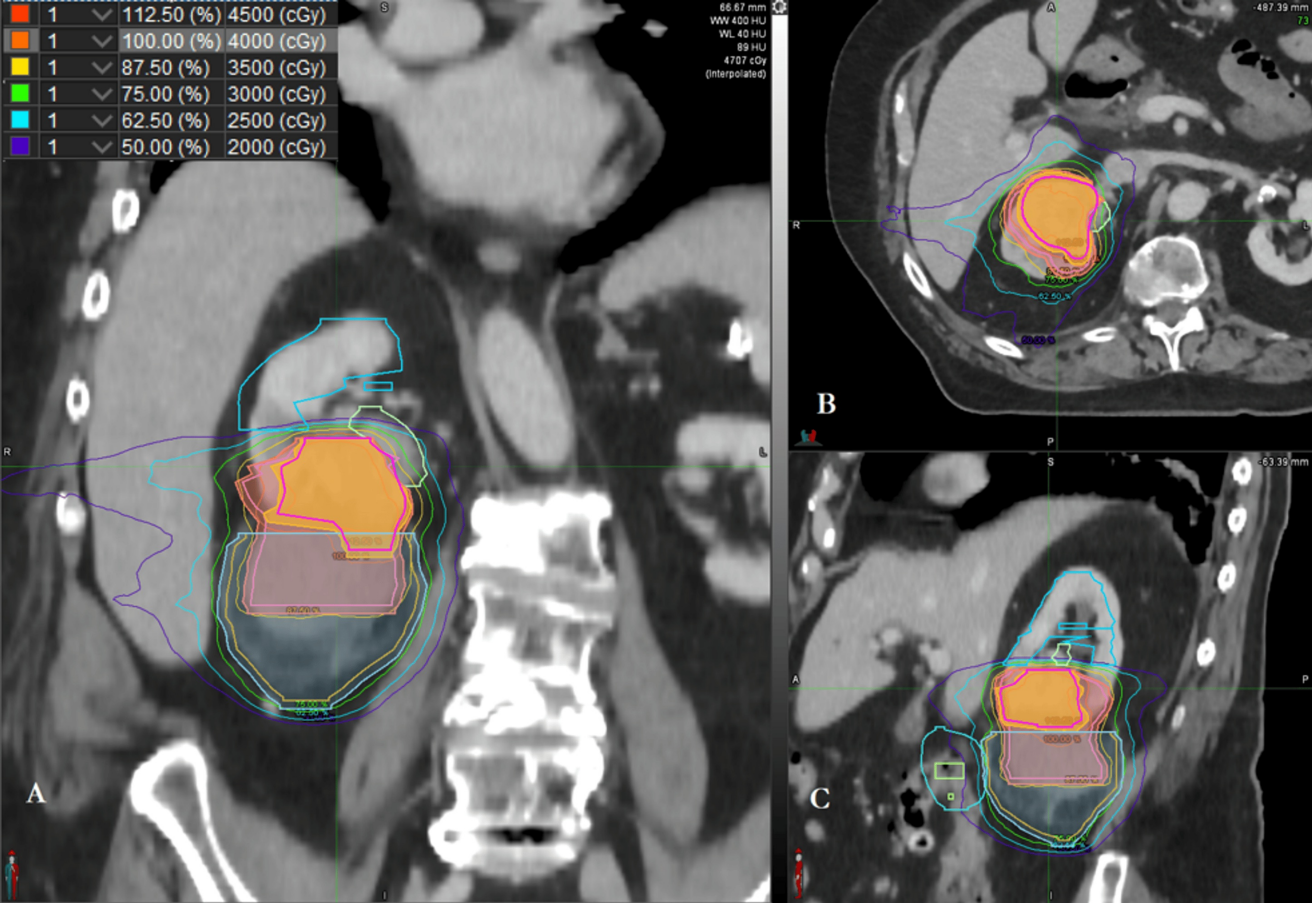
Right renal SBRT isodose maps from treatment planning CT scan shown in the (A) coronal, (B) axial, and (C) sagittal planes.
